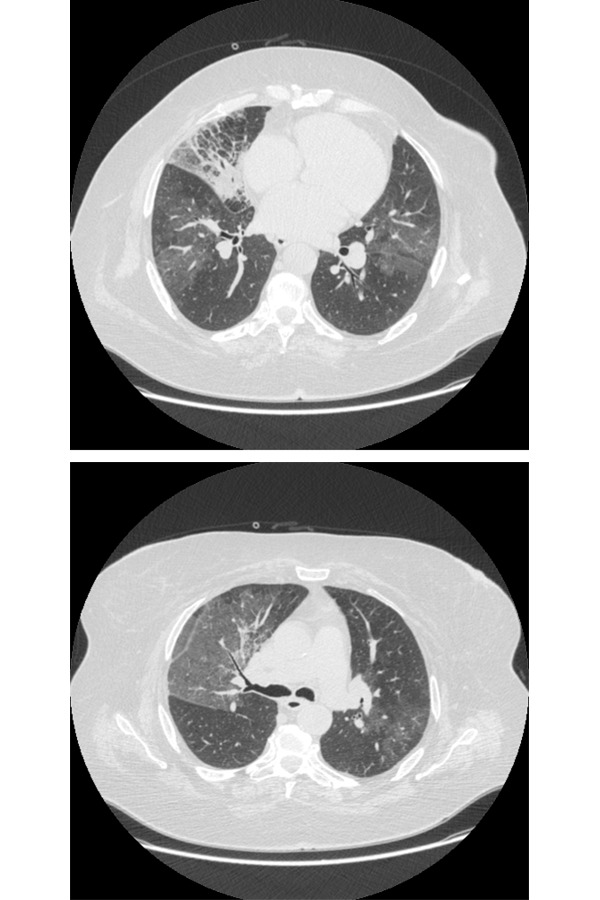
HRCT scan. Multiple extended GGO areas in both lung with middle lobe consolidation.
